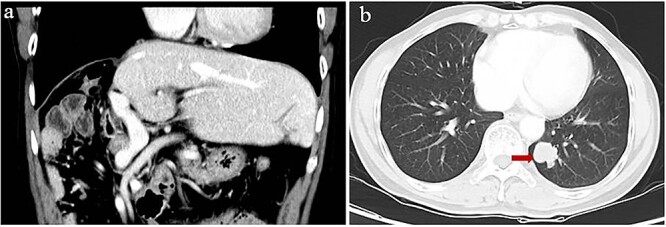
Computed tomography of lung metastasis after 2 years of adjuvant ICI. (a) Imaging at the venous stage did not reveal obvious lesions in the residual liver. (b) Imaging of the left lower lung revealed a single tumor (arrow).
